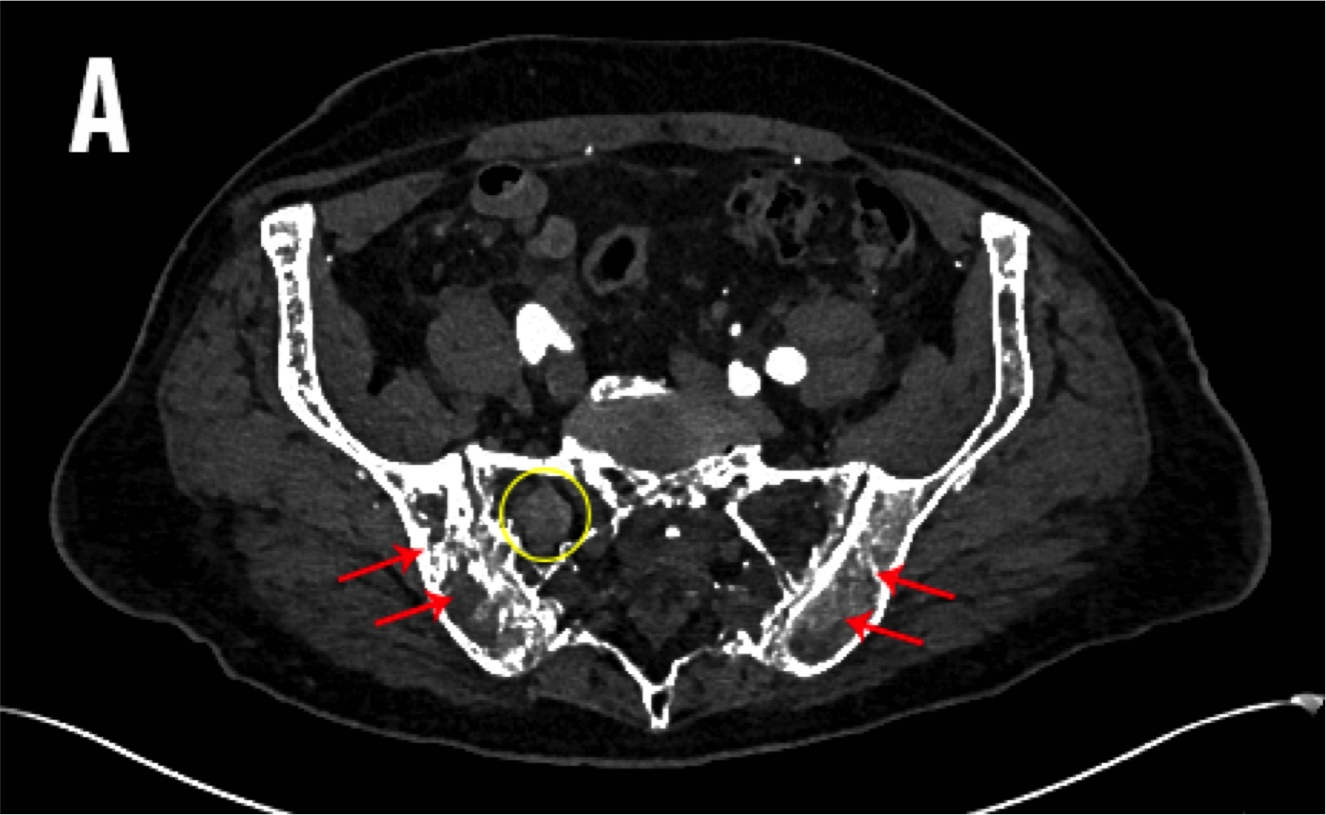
An Axial CT of Pagetic Bone Containing a Metastasis An axial contrast-enhanced CT through the pelvis: characteristic pagetic bone observed within the pelvis and sacrum (red arrows). Also seen is an enhancing mass in the upper right sacrum compatible with metastatic renal cell carcinoma (yellow circle).
